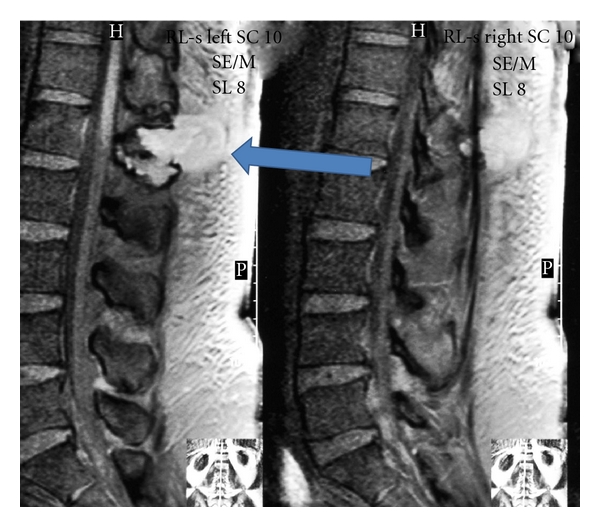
MRI of lumbar spine showing abscess at L1 (arrow).
